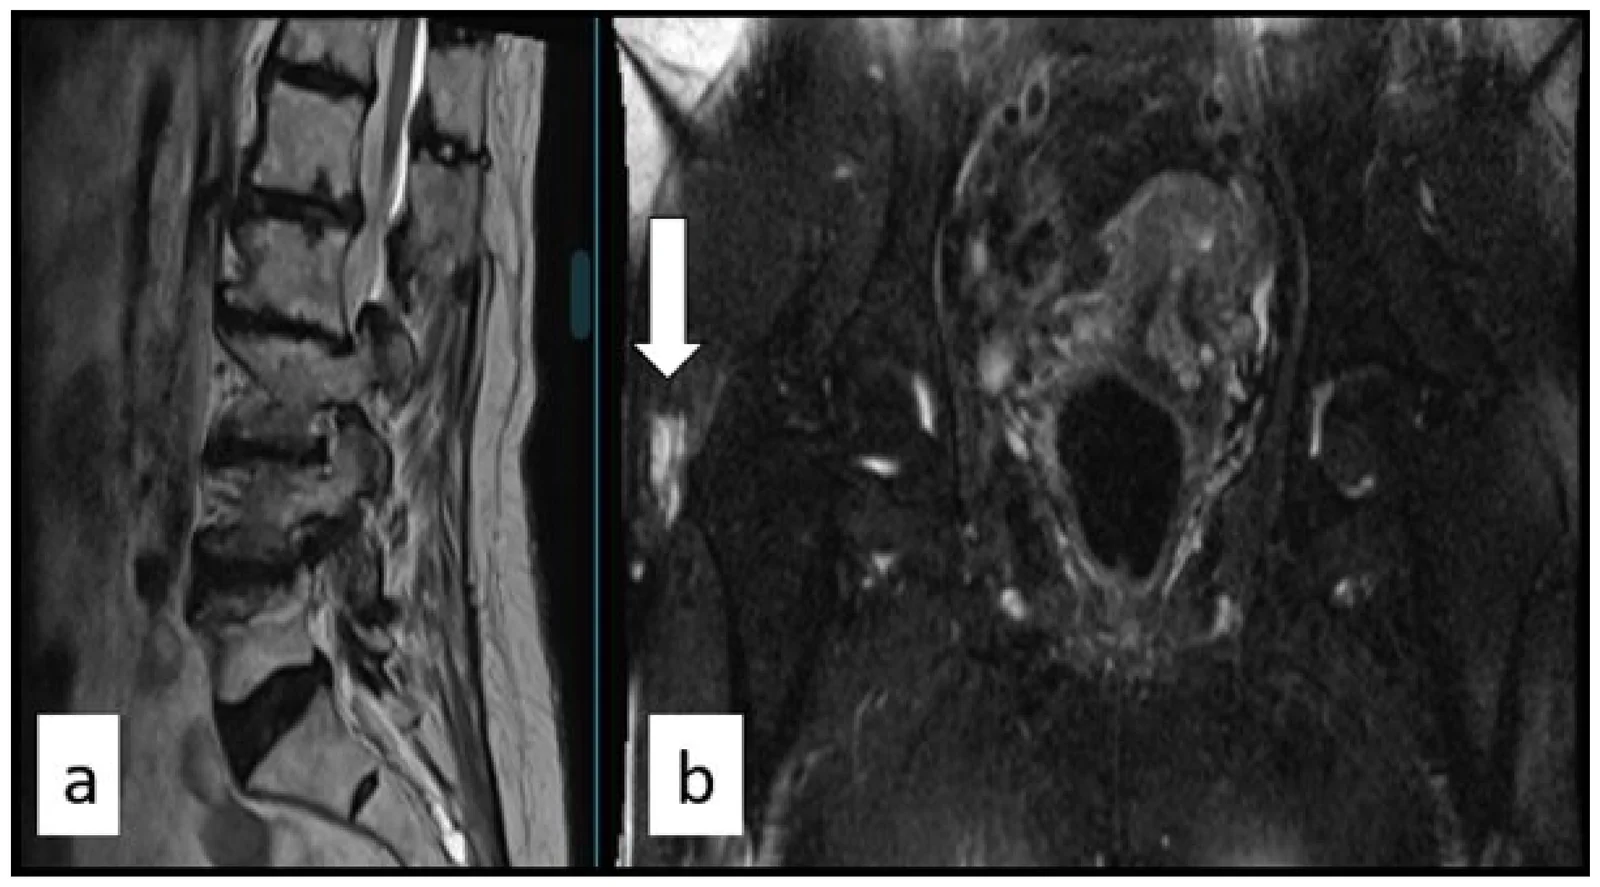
Representative anonymized MRI images from two different participants. (a) Sagittal T2-weighted lumbar MRI demonstrating degenerative spondylosis with right-sided foraminal stenosis at L3–4 and L4–5. (b) Coronal T2-weighted hip MRI from a different participant demonstrating right peritrochanteric hyperintensity consistent with trochanteric bursitis (white arrow). The panels are representative examples and were not used for patient-level lumbar–hip laterality analysis.
